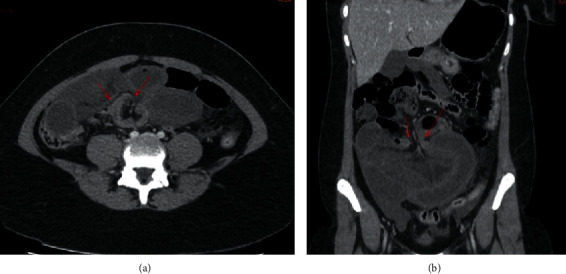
Abdominal CT scan injected in the late phase, showing a fluid dilation of the small intestine and a short junction syndrome in the periumbilical area with no image of “small bowel feces” upstream, reflecting a volvulus, with thick and poorly enhanced digestive walls: (a) axial section; (b) frontal reconstruction.
